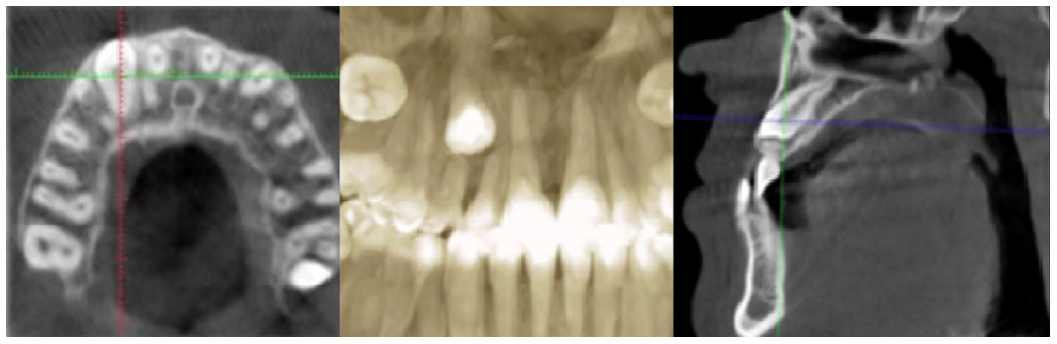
Pretreatment (CBCT) scans.
